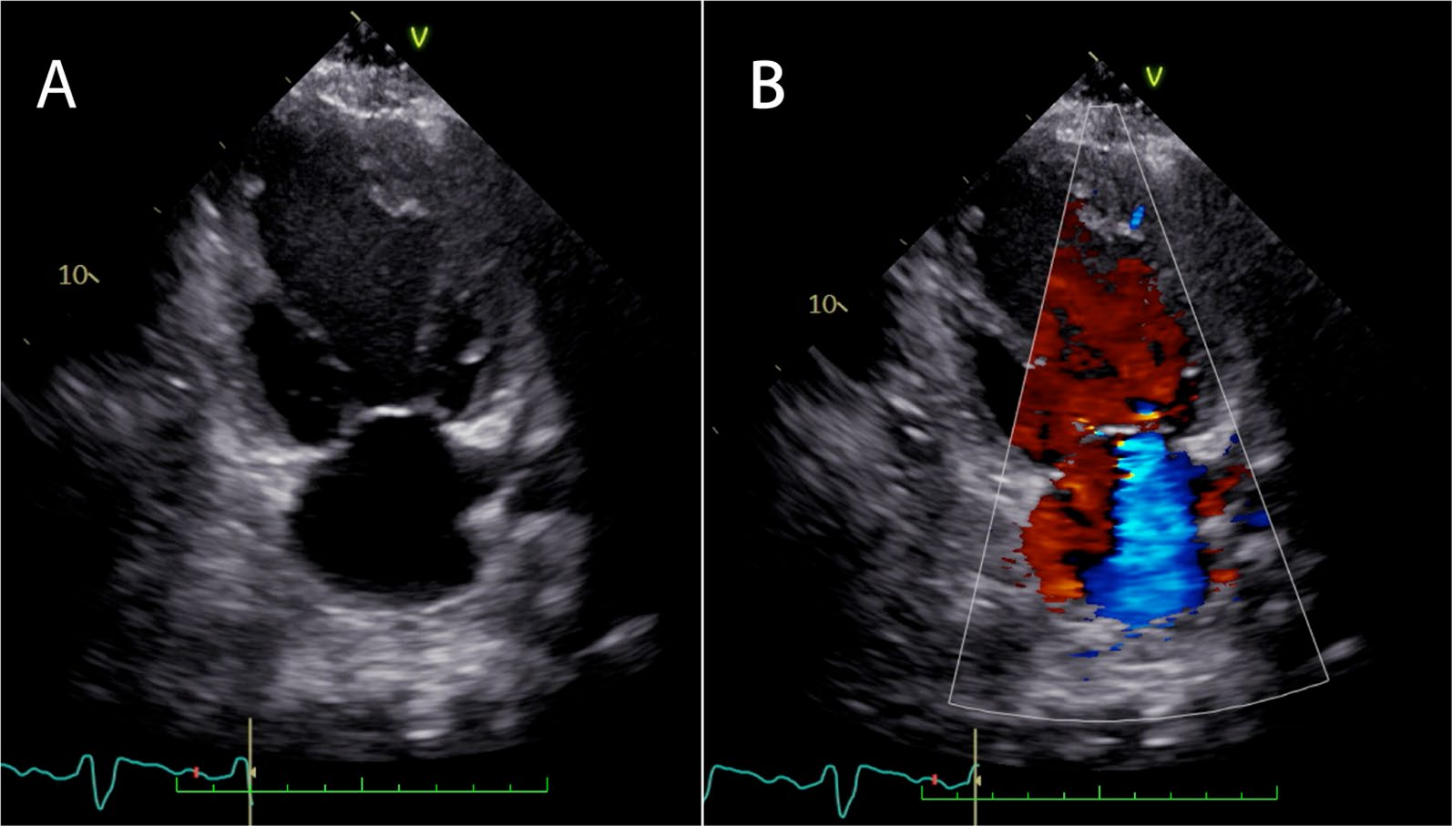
Preoperative echocardiogram. A Biplane mode of early systolic phase. B Severe mitral valve regurgitation was detected
